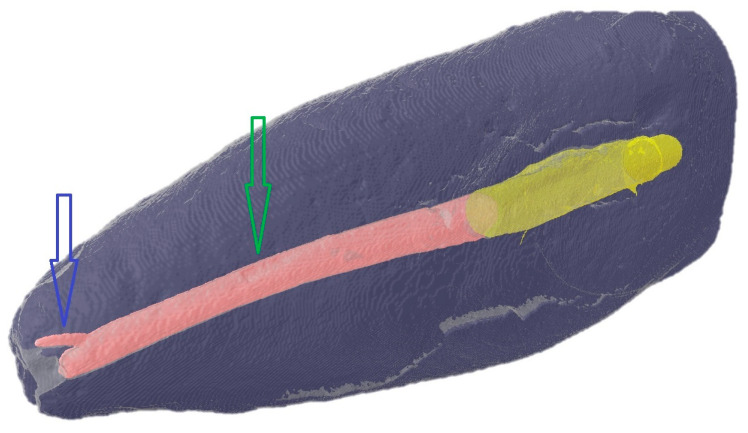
Correct apical seal (blue arrow) and canalar seal (green arrow) on a CT-Scan. Yellow: coronal third of the root canal; pink: apical and middle third of the root canal.
